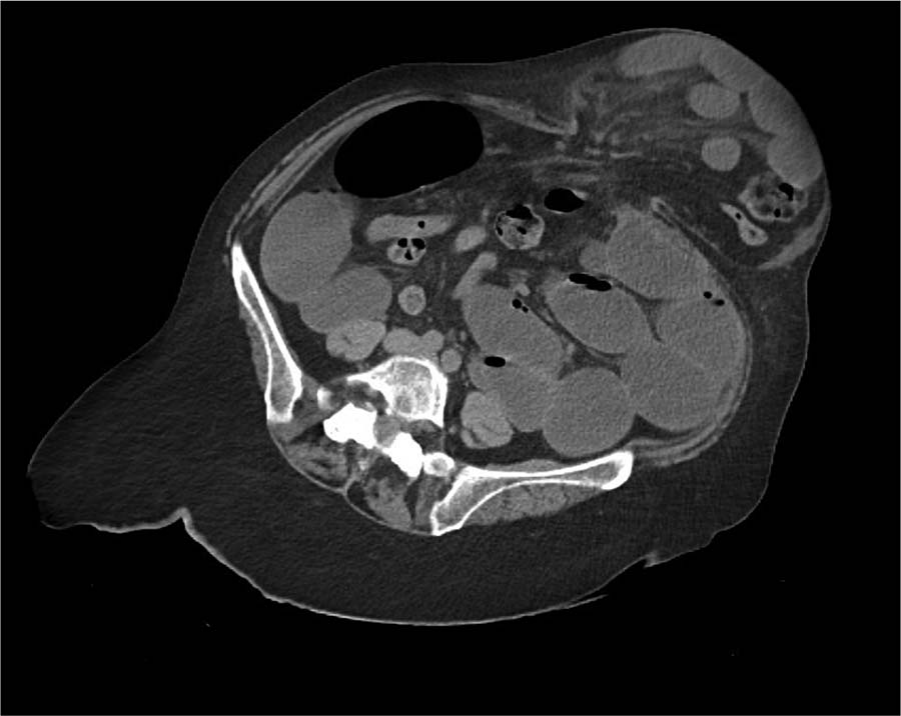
CT axial view—obstructed ventral hernia with proximal small bowel loop dilatation.
